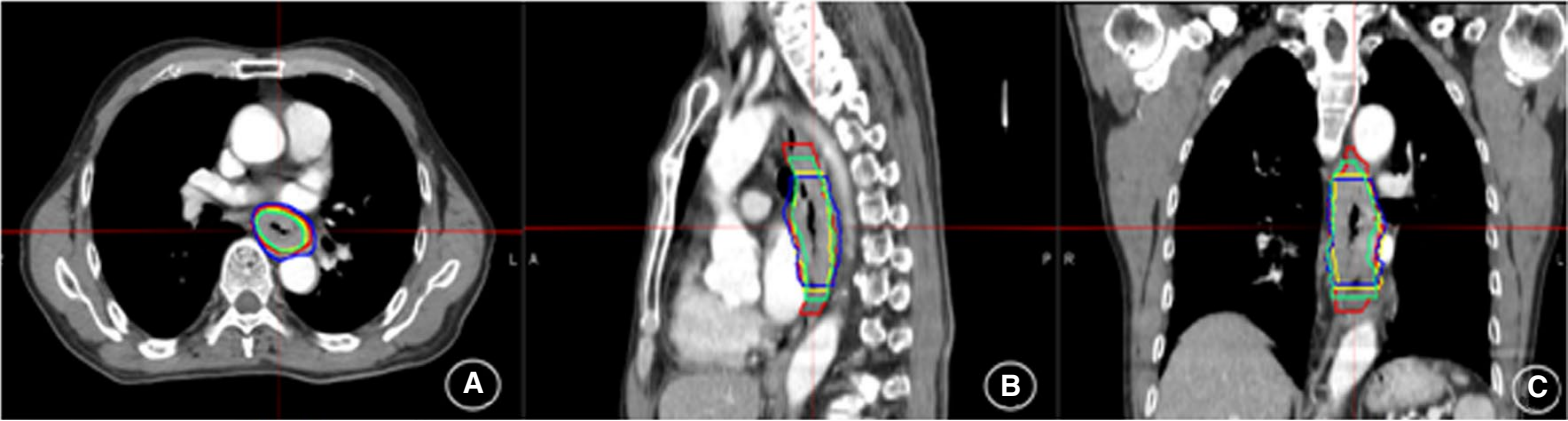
The picture of gross target volumes delineated on 3DCT(red), the EE phase of 4DCT(green), PET‐CT by the thresholds of SUV ≥ 2.5(blue), and T2W‐MRI(yellow) on transversal (A), sagittal (B), and coronal (C) in thoracic esophageal cancer
